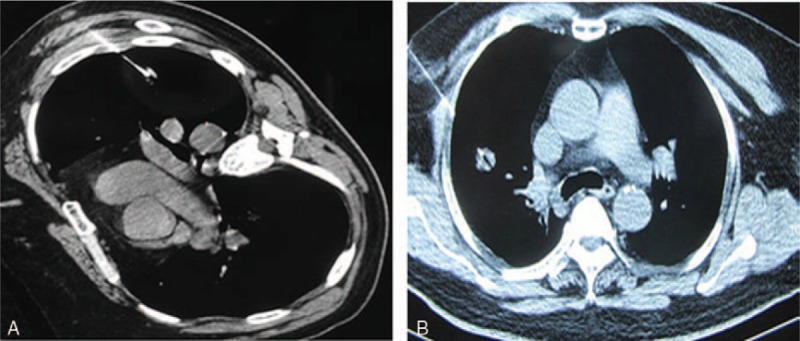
(A) CT-PTNB of the SPN lesion equal to 10 mm. (B) CT-PTNB of the SPN lesion equal to 18 mm. CT-PTNB = CT-guided percutaneous transthoracic needle biopsy, SPN = solitary pulmonary nodules.
